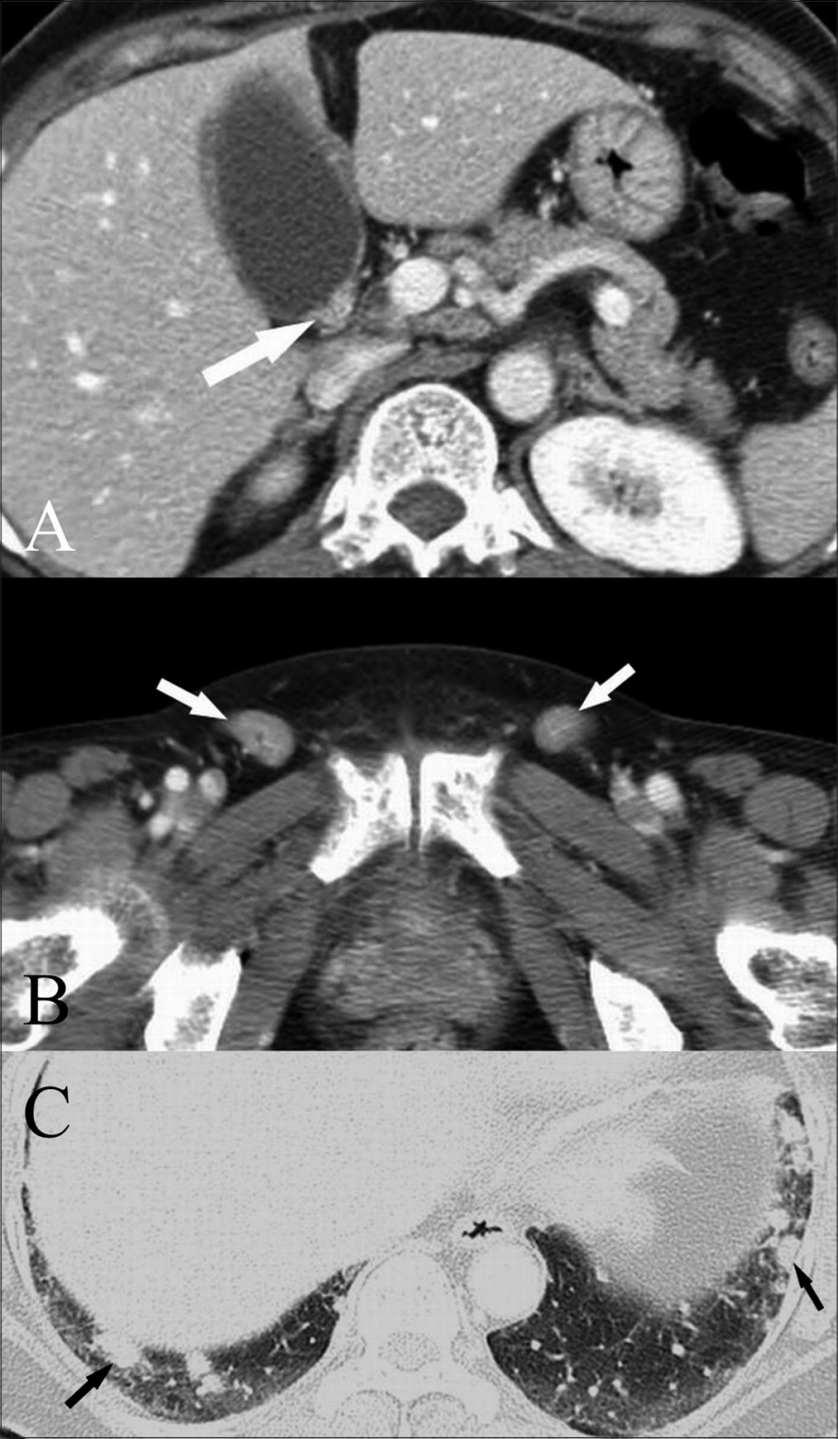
Contrast-enhanced CT scan of the abdomen (A) and pelvis (B) shows irregular wall thickening in the neck of the gallbladder (arrow in A), with multiple, enlarged inguinal lymph nodes (arrows in B) showing relatively preserved fatty hila. CT scan of the chest (C) shows multiple, well-defined, small, subcentimetre nodules (arrows), in both lower lobes
